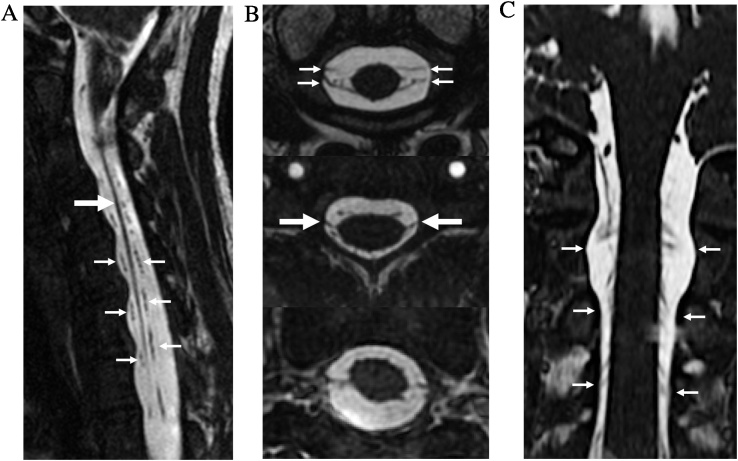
Normal Anatomy. Sagittal FIESTA-C MRI image (a) and axial and coronal reformatted images (b–c) demonstrate the detailed intradural anatomy of the cervical spine, including the denticulate ligament (large arrows in a–b), as well as the paired ventral and dorsal rootlets of the spinal nerves (small arrows in a–c).
